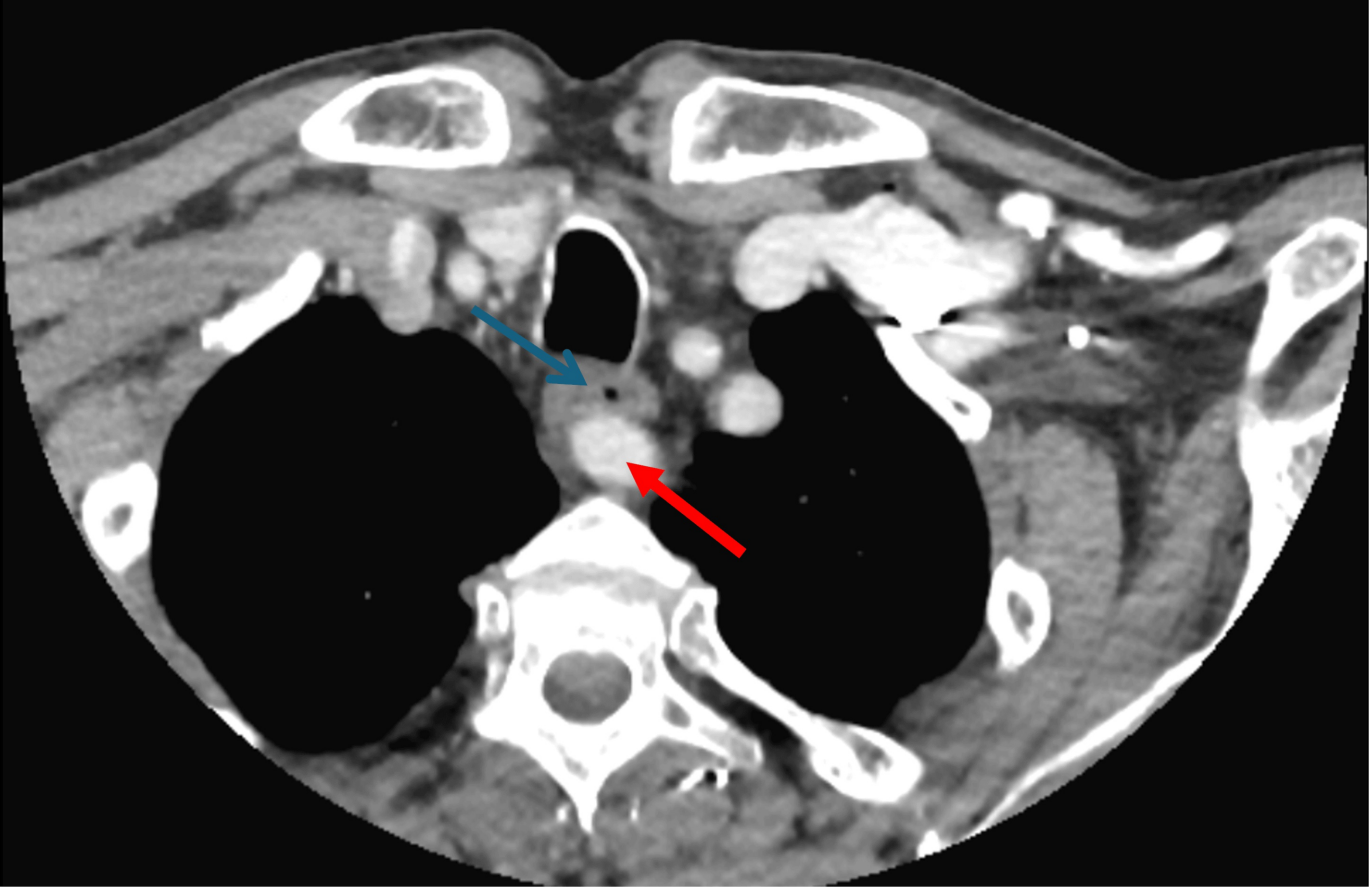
Axial view of the CT head and neck with the aberrant right subclavian artery (red arrow) overriding the esophagus (blue arrow).
